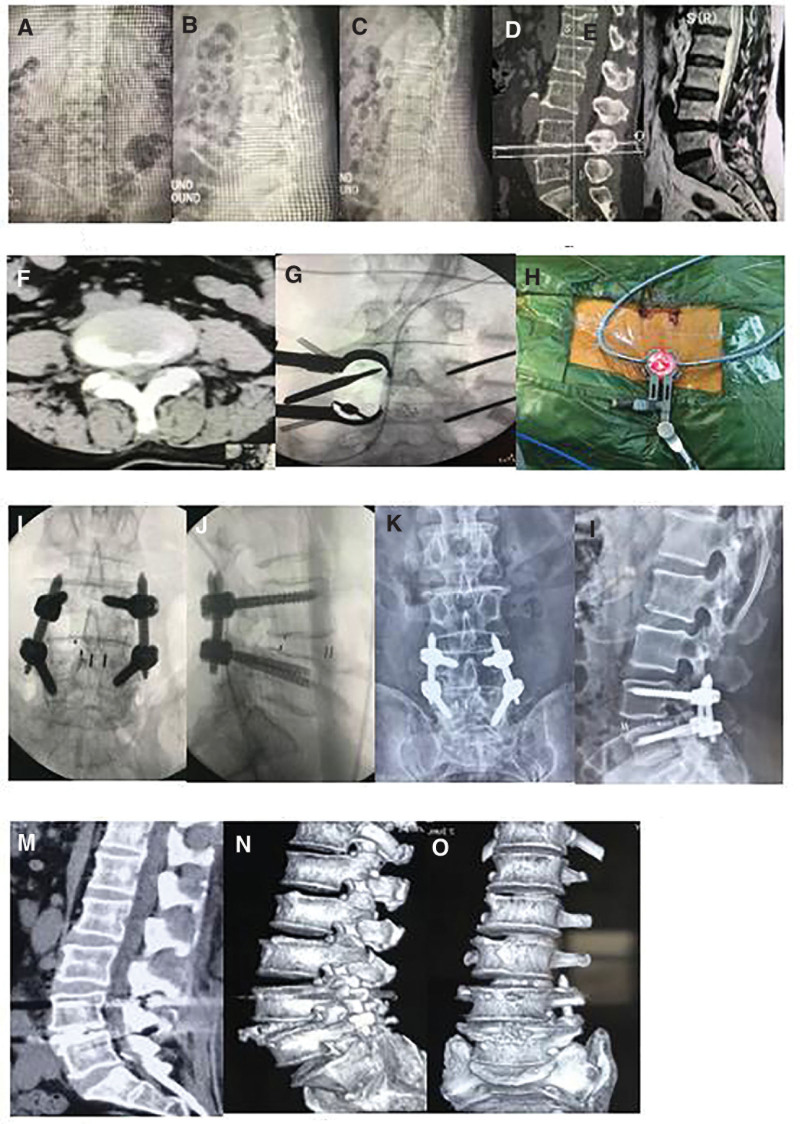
The patient was a 57 year old female, had recurrent low back pain with radiation pain of the right lower limb for 1 year and aggravated for 2 months. Her preoperative imaging examination (A–F), and She chosed MIS-TLIF operation (G, H). She underwent X-ray examination after operation (I, J) and reviewed in 3 (K, L) and 6 (M) months after operation, and CT 3-dimensional reconstruction in 6 months after operation (N, O). MIS-TLIF = minimally invasive transforaminal lumbar interbody fusion.
